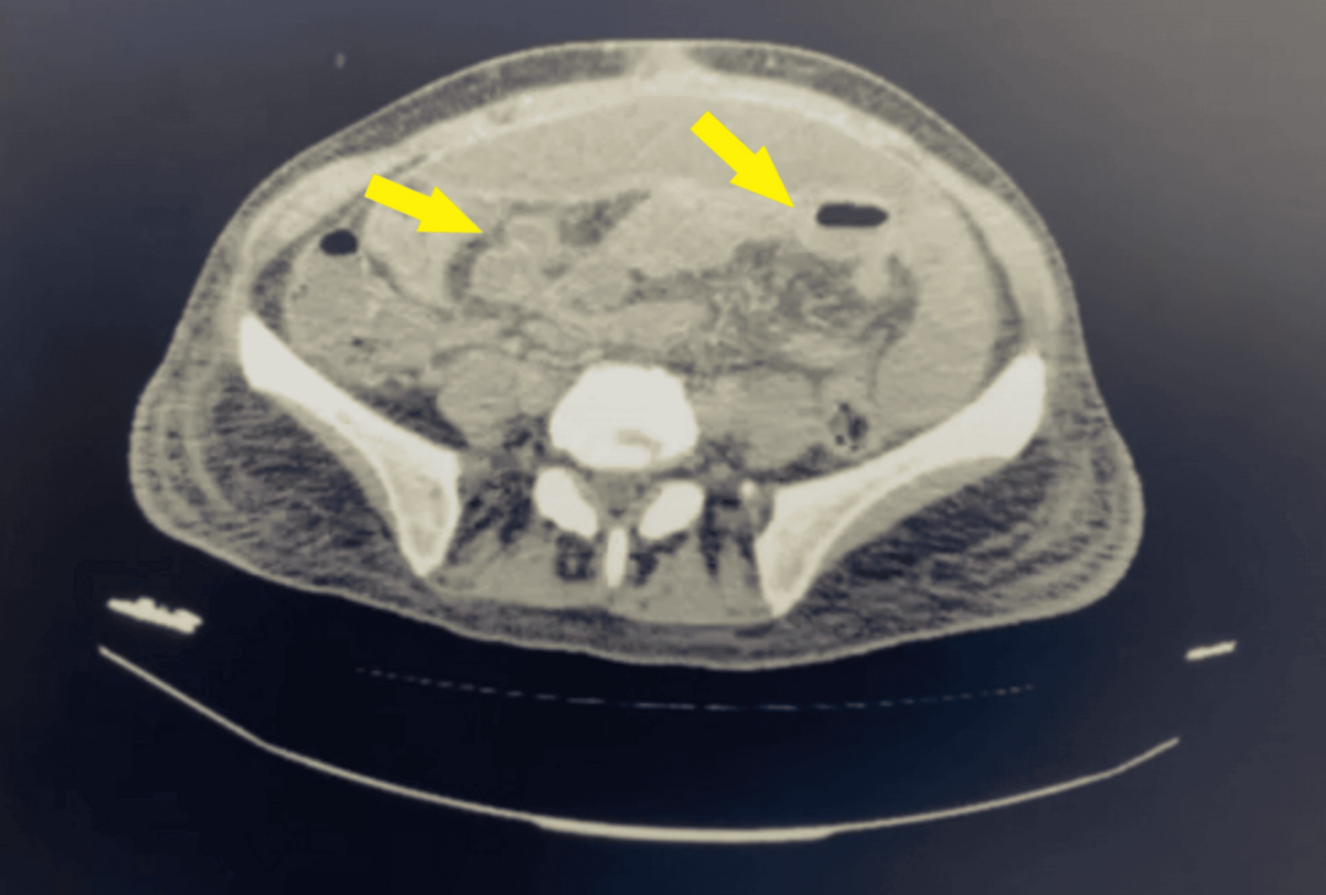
CT scan performed after the initial conservative treatment revealed an improvement in small intestine dilation (yellow arrows).
